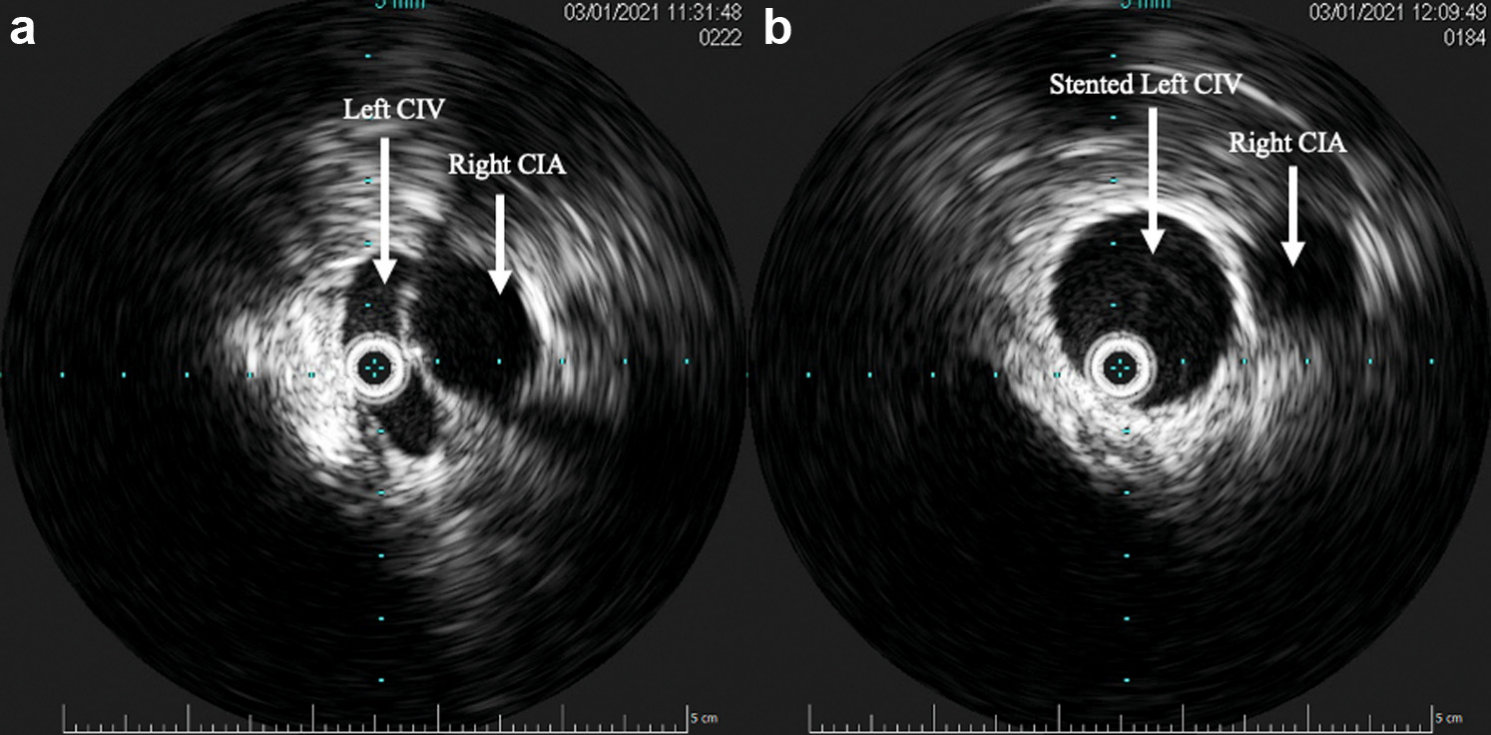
Preintervention intravascular ultrasound examination showing compression of the left common iliac vein (CIV) by the right common iliac artery (A) with resolution of compression after stenting (B).
